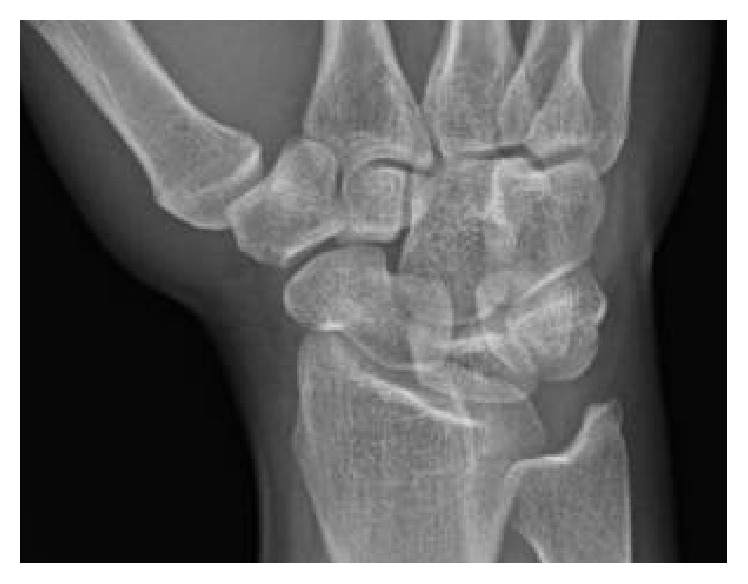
An oblique radiographic view at the time of initial evaluation without evidence of abnormality of the scaphoid.
